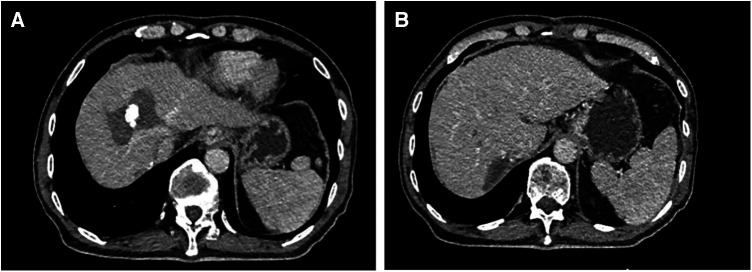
Postoperative CT images of the patient. (A) The CT image of the lesion in segment VIII after RFA. (B) The main HCC mainly in segment VII was resected after systemic treatment combined with TACE treatment.
